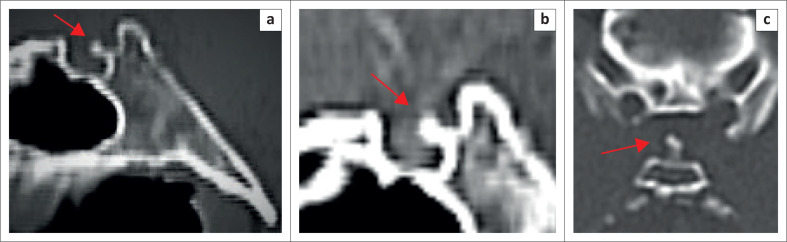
CT of the pituitary fossa. (a) Bone window in the sagittal plane in the midline demonstrating the sellar spine (arrow) protruding into the pituitary fossa producing a ‘figure of 3’ sign of the dorsum sellae. (b) Soft tissue window in the sagittal plane in the midline demonstrating compression of the pituitary and distal pituitary stalk by the sellar spine (arrow). (c) Bone window in the axial plane demonstrating the midline position of the sellar spine (arrow).
